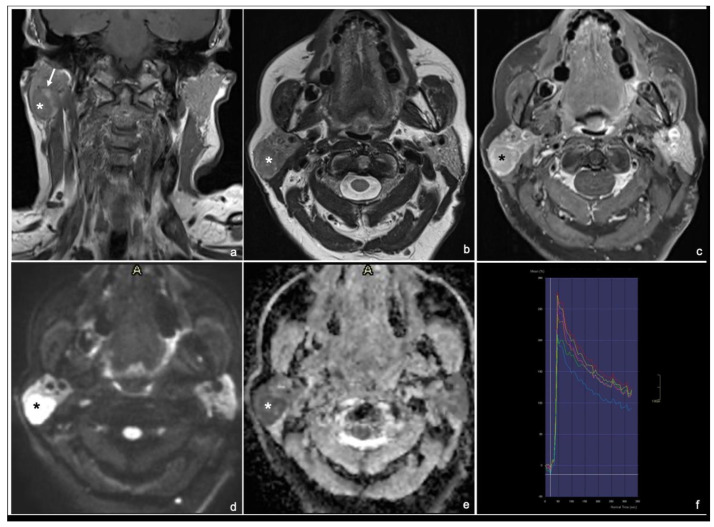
65 years old woman with Warthin tumor of the right parotid gland: coronal T1 TSE (a), axial T2 TSE (b), late T1 TSE FS + Gd (c), DWI and ADC map (d,e) and DCE signal intensity-time S(t)-curve type B (f). * tumor. The lesion is characterized by T1 hyperintense intratumoral cysts (arrow) (a) and low enhancement on late T1 post-contrast sequence (normalized late T1 + Gd SI < 2) with isointensity to the normal parotid tissue with a thin peripheral rim enhancement (“ghosting sign”) (c).
